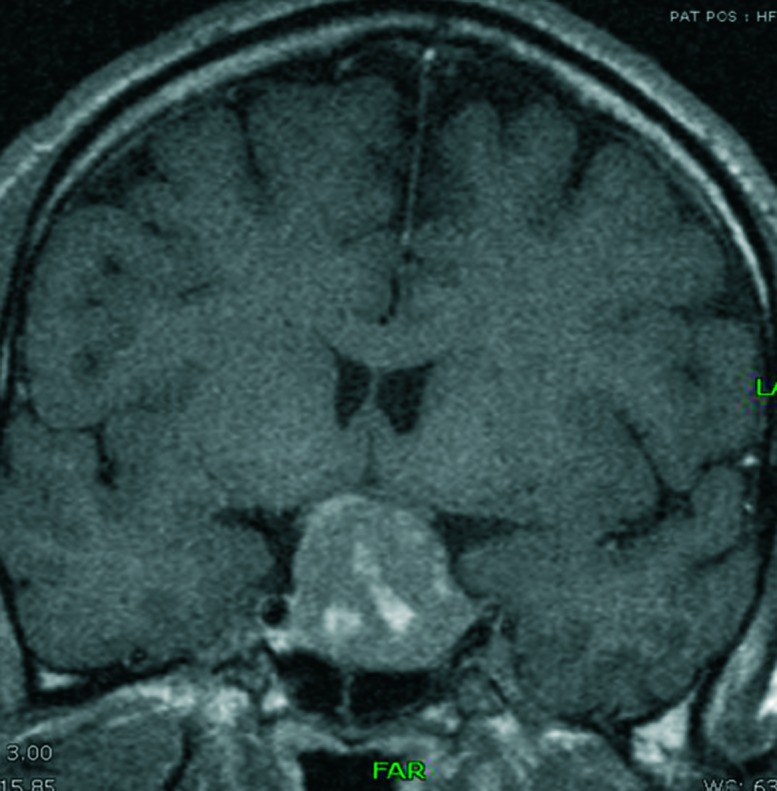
MRI scan showing a pituitary macro-adenoma with supra- and parasellar extension.
